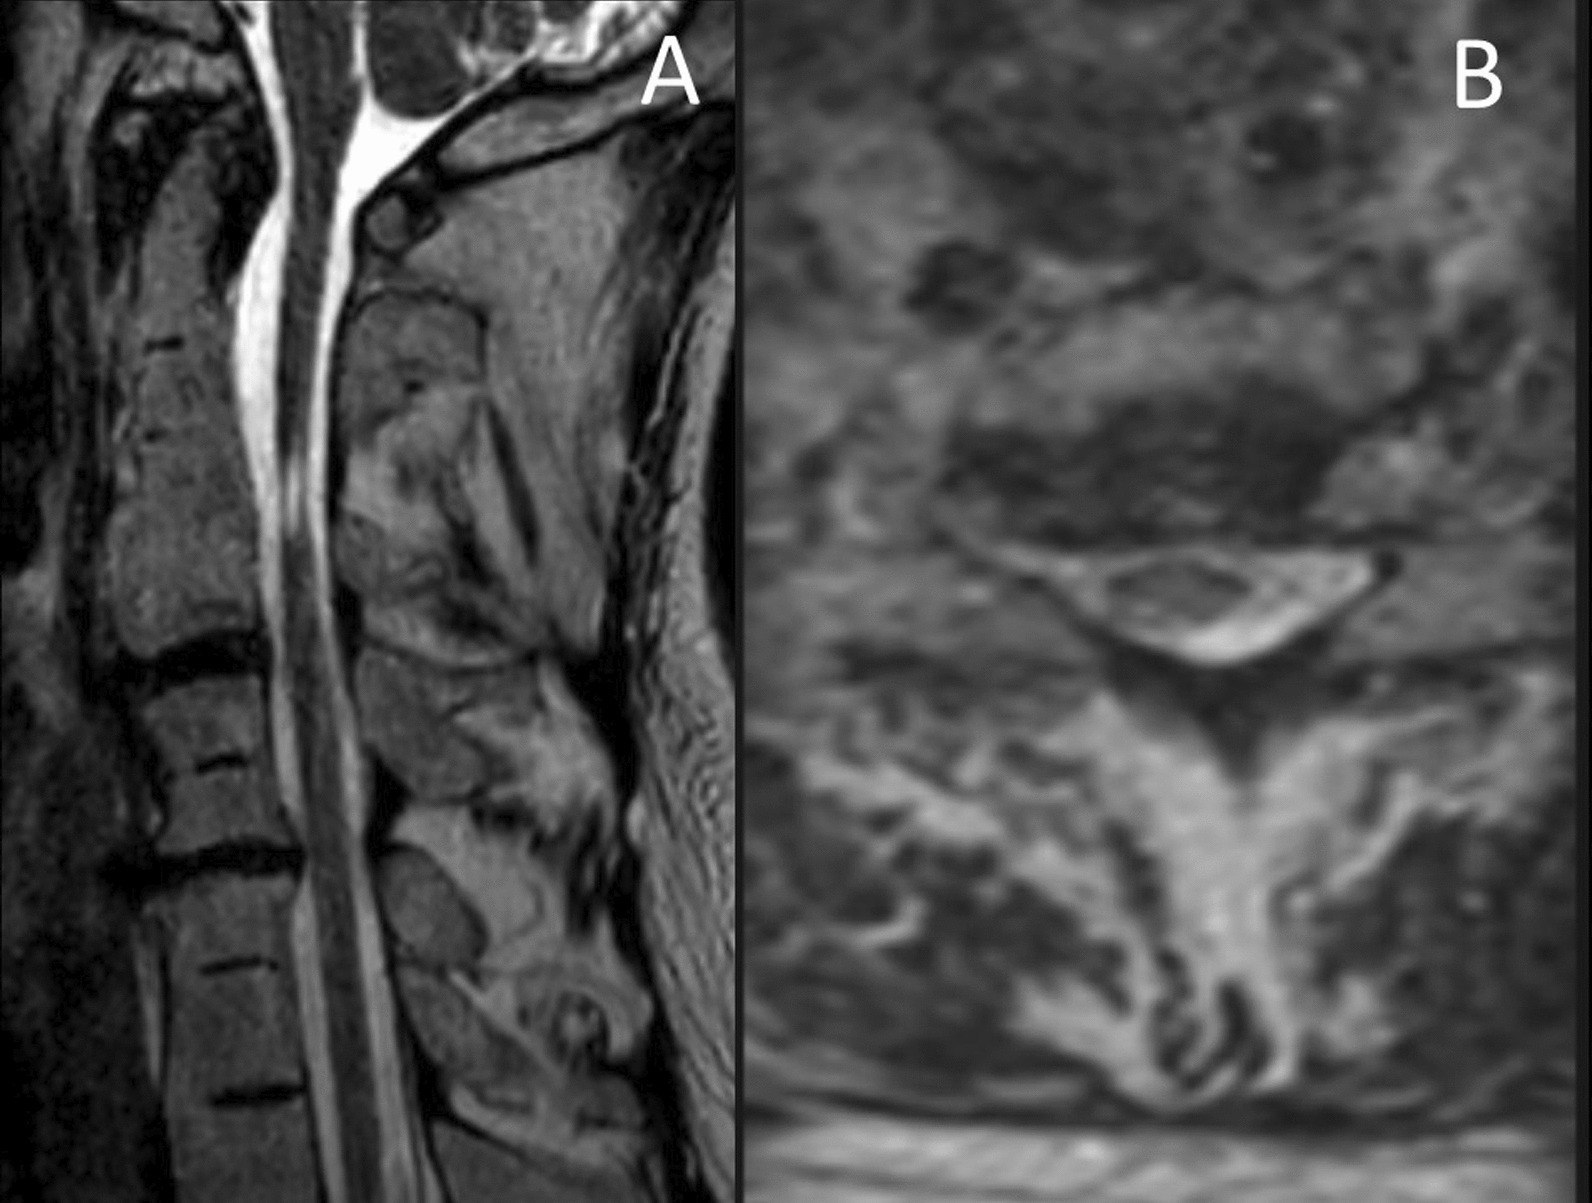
Cervical spine T2-sequence magnetic resonance imaging. A sagittal view showing the canal stenosis at C6–C7, as well as the hypersignal at the level of C3. B axial view of the C6–C7 level which shows a moderately stenotic cervical canal
